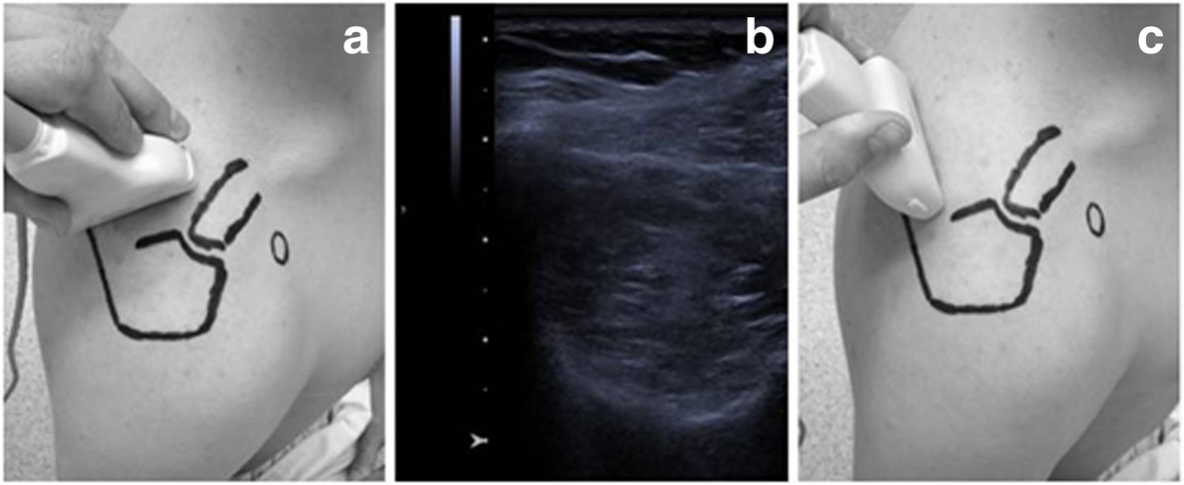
Experimental setting. The ultrasound probe was placed in an oblique sagittal position (a). The largest diameter of the supraspinatus muscles was detected using b-mode ultrasound (b). SWE measurement was performed at this location in a parallel orientation of the transducer to the muscle fibers (c). The velocity of shear wave propagation was measured at 10–15 points in the ROI. The measurement was repeated 5 times to cover almost the whole cross section area of the muscle. To ensure spectroscopic measurement at the same location the point of measurement was marked with a MRI visible gel capsule fixed to the patient’s skin
